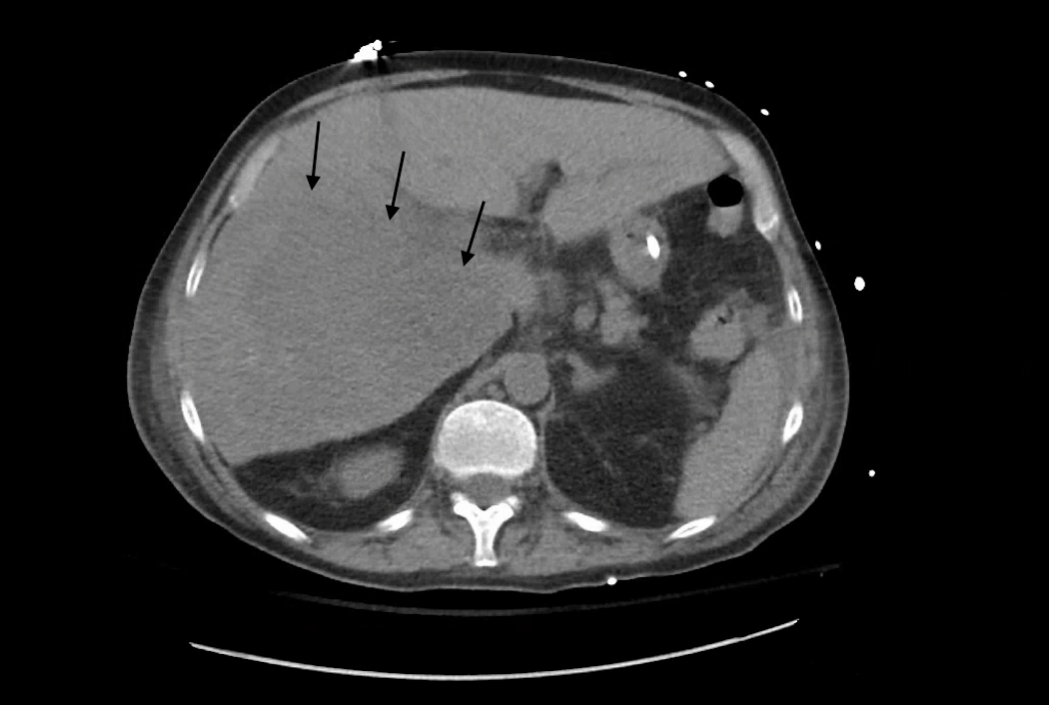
Non-Contrasted Computed Tomography of Abdomen Non-contrasted computed tomography of abdomen performed on hospital day 14 demonstrates increased area of hypoattenuation within the right hepatic lobe (arrows).
